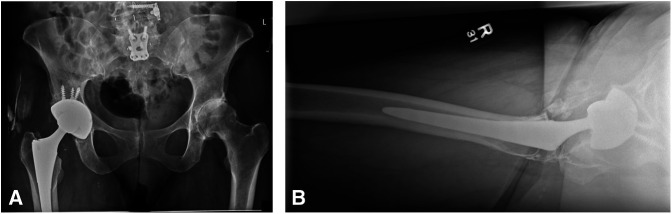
A, Postoperative AP pelvis radiograph after revision surgery showing the dual-mobility implants and (B) the postoperative lateral radiograph.
